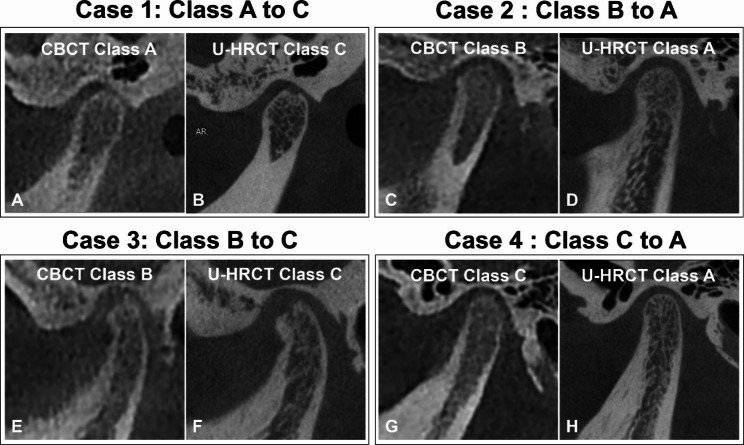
Discrepant diagnoses by CBCT and U-HRCT. (A-B) Left TMJ of a 41-year-old woman who is assigned Class A (continuous cortical bone of the condyle without erosion) on CBCT but Class C (bony erosion on the roof of the condyle) on U-HRCT. (C-D) Right TMJ of a 16-year-old man who is assigned Class B (suspicious discontinuity on the posterior border of the condyle) on CBCT but Class A (no evident bony changes) on U-HRCT. (E-F) Left TMJ of a 20-year-old woman who is assigned Class B (suspicious discontinuity on the posterior border of the condyle and osteophyte on the roof) on CBCT but Class C (bony erosion and osteophyte of the condyle) on U-HRCT. (G-H) Right TMJ of a 16-year-old woman who is assigned Class C (suspicious bony discontinuity on the posterior border of the condyle) on CBCT but Class A (no evident bony changes) on U-HRCT.
